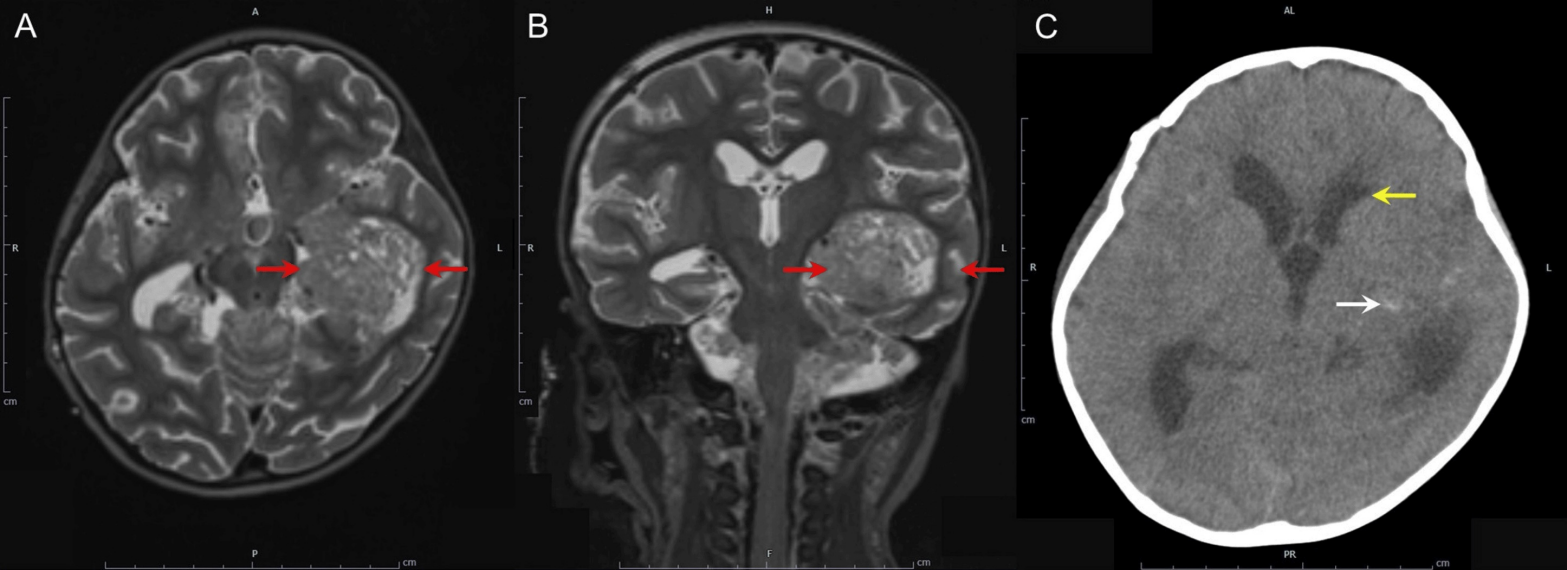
MR FLAIR and CT images of the brain in Case 1. Axial (A) and coronal (B) MR FLAIR images showing a mass lesion with mild heterogeneous hyperintensity in the left temporal lobe and lateral ventricle (red arrow); (C) CT image showing narrowing of the lateral ventricles (yellow arrow) and intratumoral hemorrhage (white arrow). MR: magnetic resonance; CT: computed tomography; FLAIR: fluid-attenuated inversion recovery
